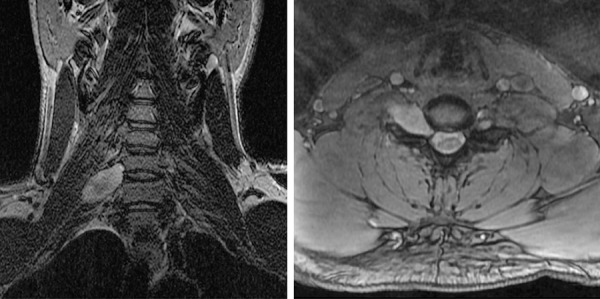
MR imaging of the cervical spine demonstrating an intradural, extramedullary mass centered in the right C6-C7 neural foramen and extending along the C7 nerve root (coronal T2-weighted, left; axial T1-weighted post-gadolinium, right).
